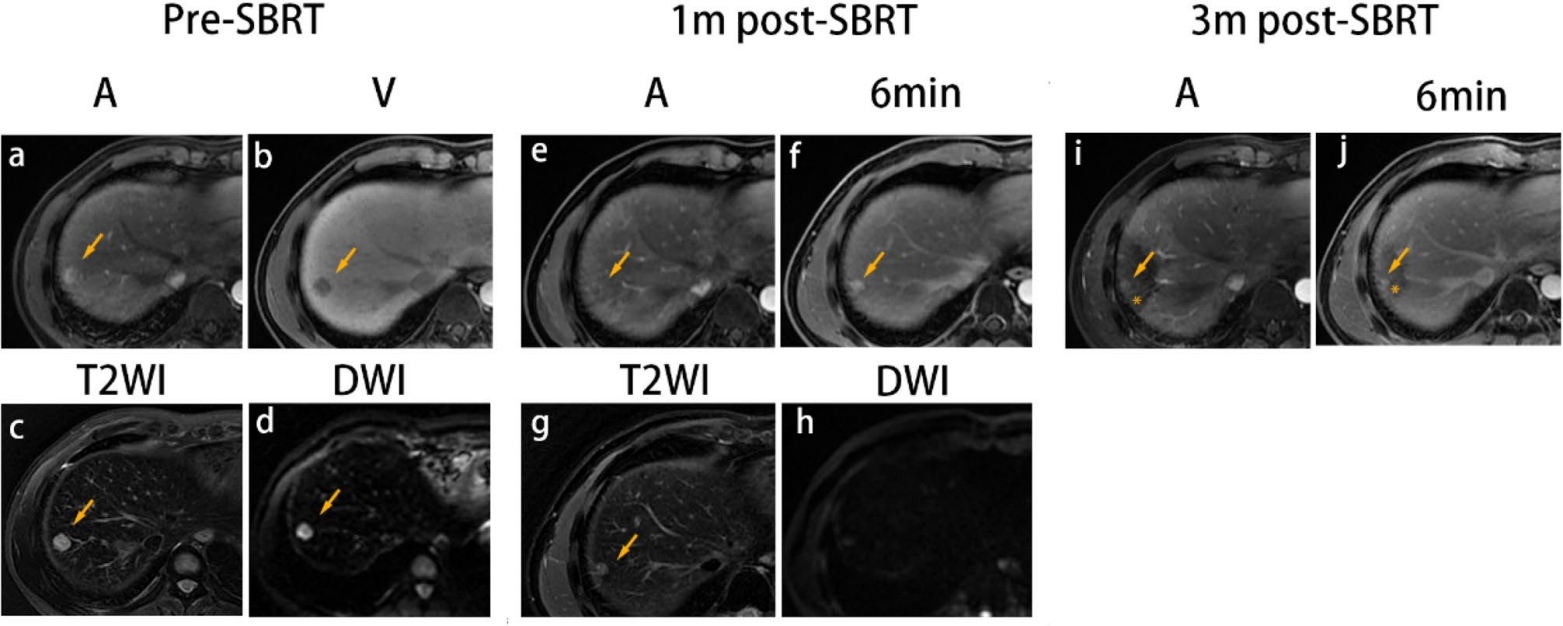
SBRT-treated HCC with conversion from APHE and washout before SBRT to delayed enhancement immediately after SBRT. A 44-year-old man with HBV-related cirrhosis and a 1.5-cm HCC in the seventh segment of the liver (arrow). At baseline, the lesion exhibited APHE and washout (a, b). Additionally, the lesion was hyperintense on T2WI (c) and DWI (d). At 1-month post-SBRT, the lesion demonstrated conversion to delayed enhancement (hypoenhancement on arterial phase imaging with increasing enhancement) (e, f) with a decrease in size, measuring 1.1 cm and a decrease in signal intensity on T2WI (g) and DWI (h). At 3 months post-SBRT, the lesion presented persistent delayed enhancement (i, j). Furthermore, at 3 months post-SBRT, there was wedge-like parenchymal arterial phase hypoenhancement and an increase in delayed phase images in the surrounding treated zone (asterisk). The lesion was categorized as CR and LR-TR nonviable based on m-RECIST and LI-RADS. *SBRT* stereotactic body radiation therapy; *A* arterial phase; *V* portal venous phase; *T2WI* T2-weighted imaging; *DWI* diffusion-weighted imaging; 6 min, delayed phase (6 min); *CR* complete response; *APHE* arterial phase hyperenhancement; *HCC* hepatocellular carcinoma; *m-RECIST* Modified Response Evaluation Criteria in Solid Tumors; *LI-RADS* Liver Reporting and Data System
